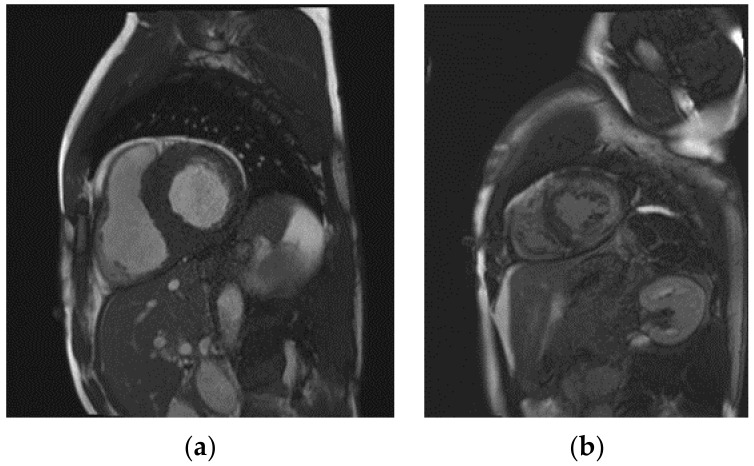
Exemplary cardiac magnetic resonance images of a Fabry disease patient with pronounced left ventricular hypertrophy (a) and an extensive area of late gadolinium enhancement (b).
